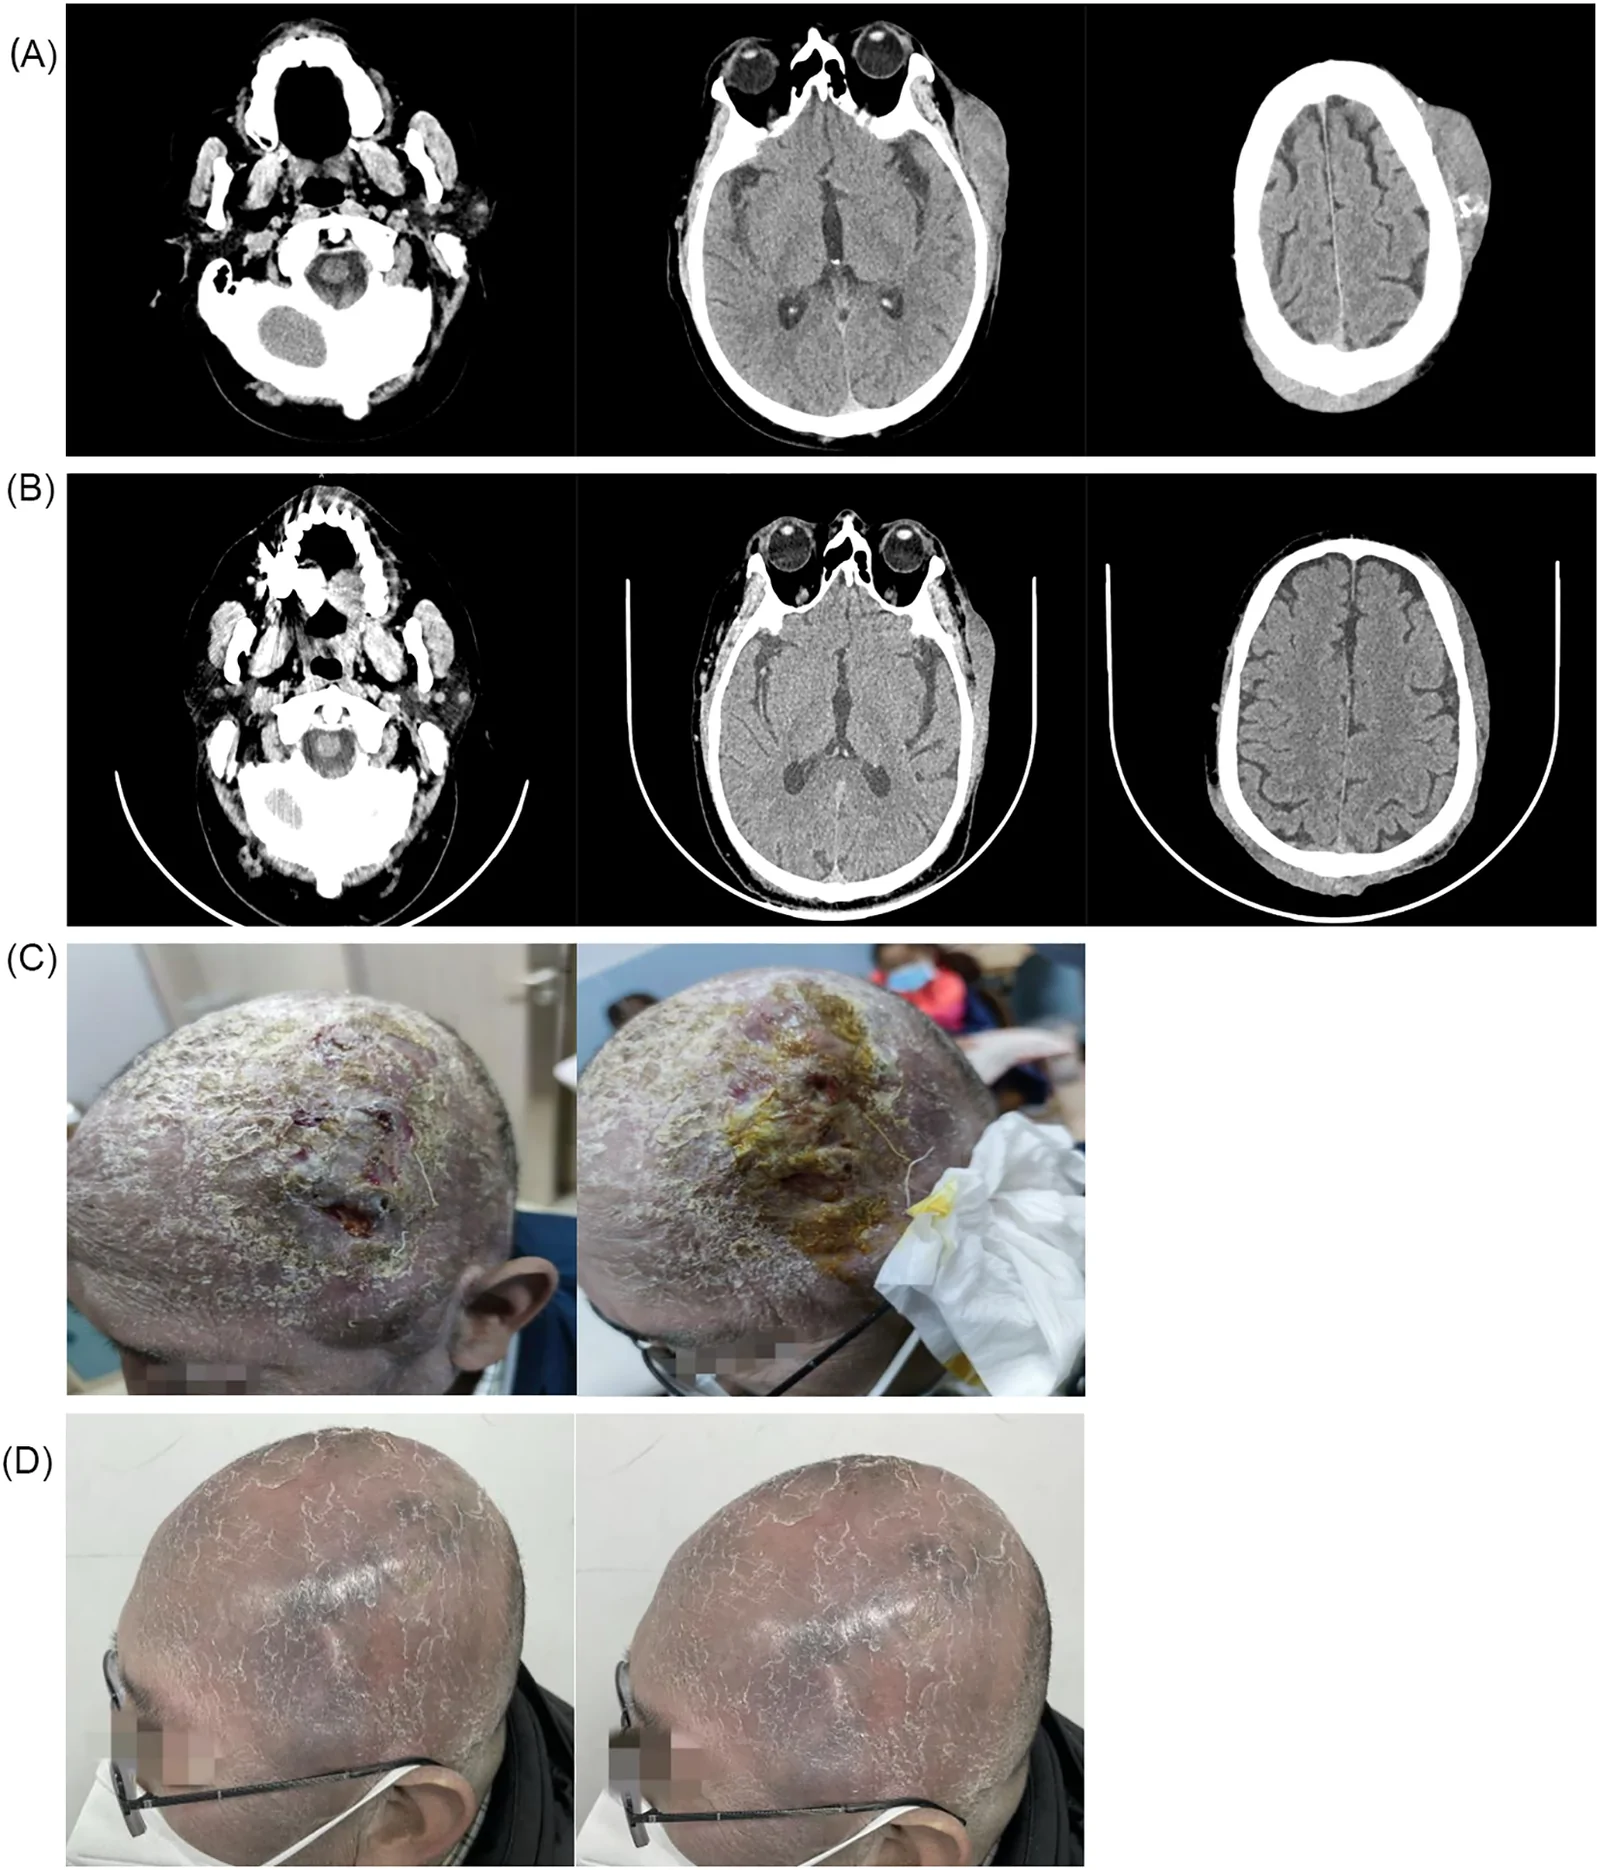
Pre- and post- treatment imaging and physical signs of patient 1. (A, C) Baseline cranial and parotid computed tomography (CT) images as well as physical signs; (B, D) cranial and parotid CT images obtained after a 2-cycle treatment, during an off-treatment follow-up period of up to 8 months, as well as physical signs. Alt text: Figure showing pre- and post-treatment imaging and clinical findings for a patient with scalp lesions. (A) Pre-treatment axial computed tomography(CT) scans of the head at three levels, revealing a well-defined, hyperdense, expansile lesion in the left parietal scalp, with associated calvarial erosion. (B) Post-treatment axial CT scans of the head at corresponding levels, demonstrating significant regression of the scalp lesion and resolution of calvarial erosion. (C) Pre-treatment clinical photographs of the patient’s scalp, showing a large, ulcerated, crusted lesion with surrounding erythema and scale. (D) Post-treatment clinical photographs of the patient’s scalp, showing complete healing of the lesion, with only residual scarring and mild desquamation remaining.
